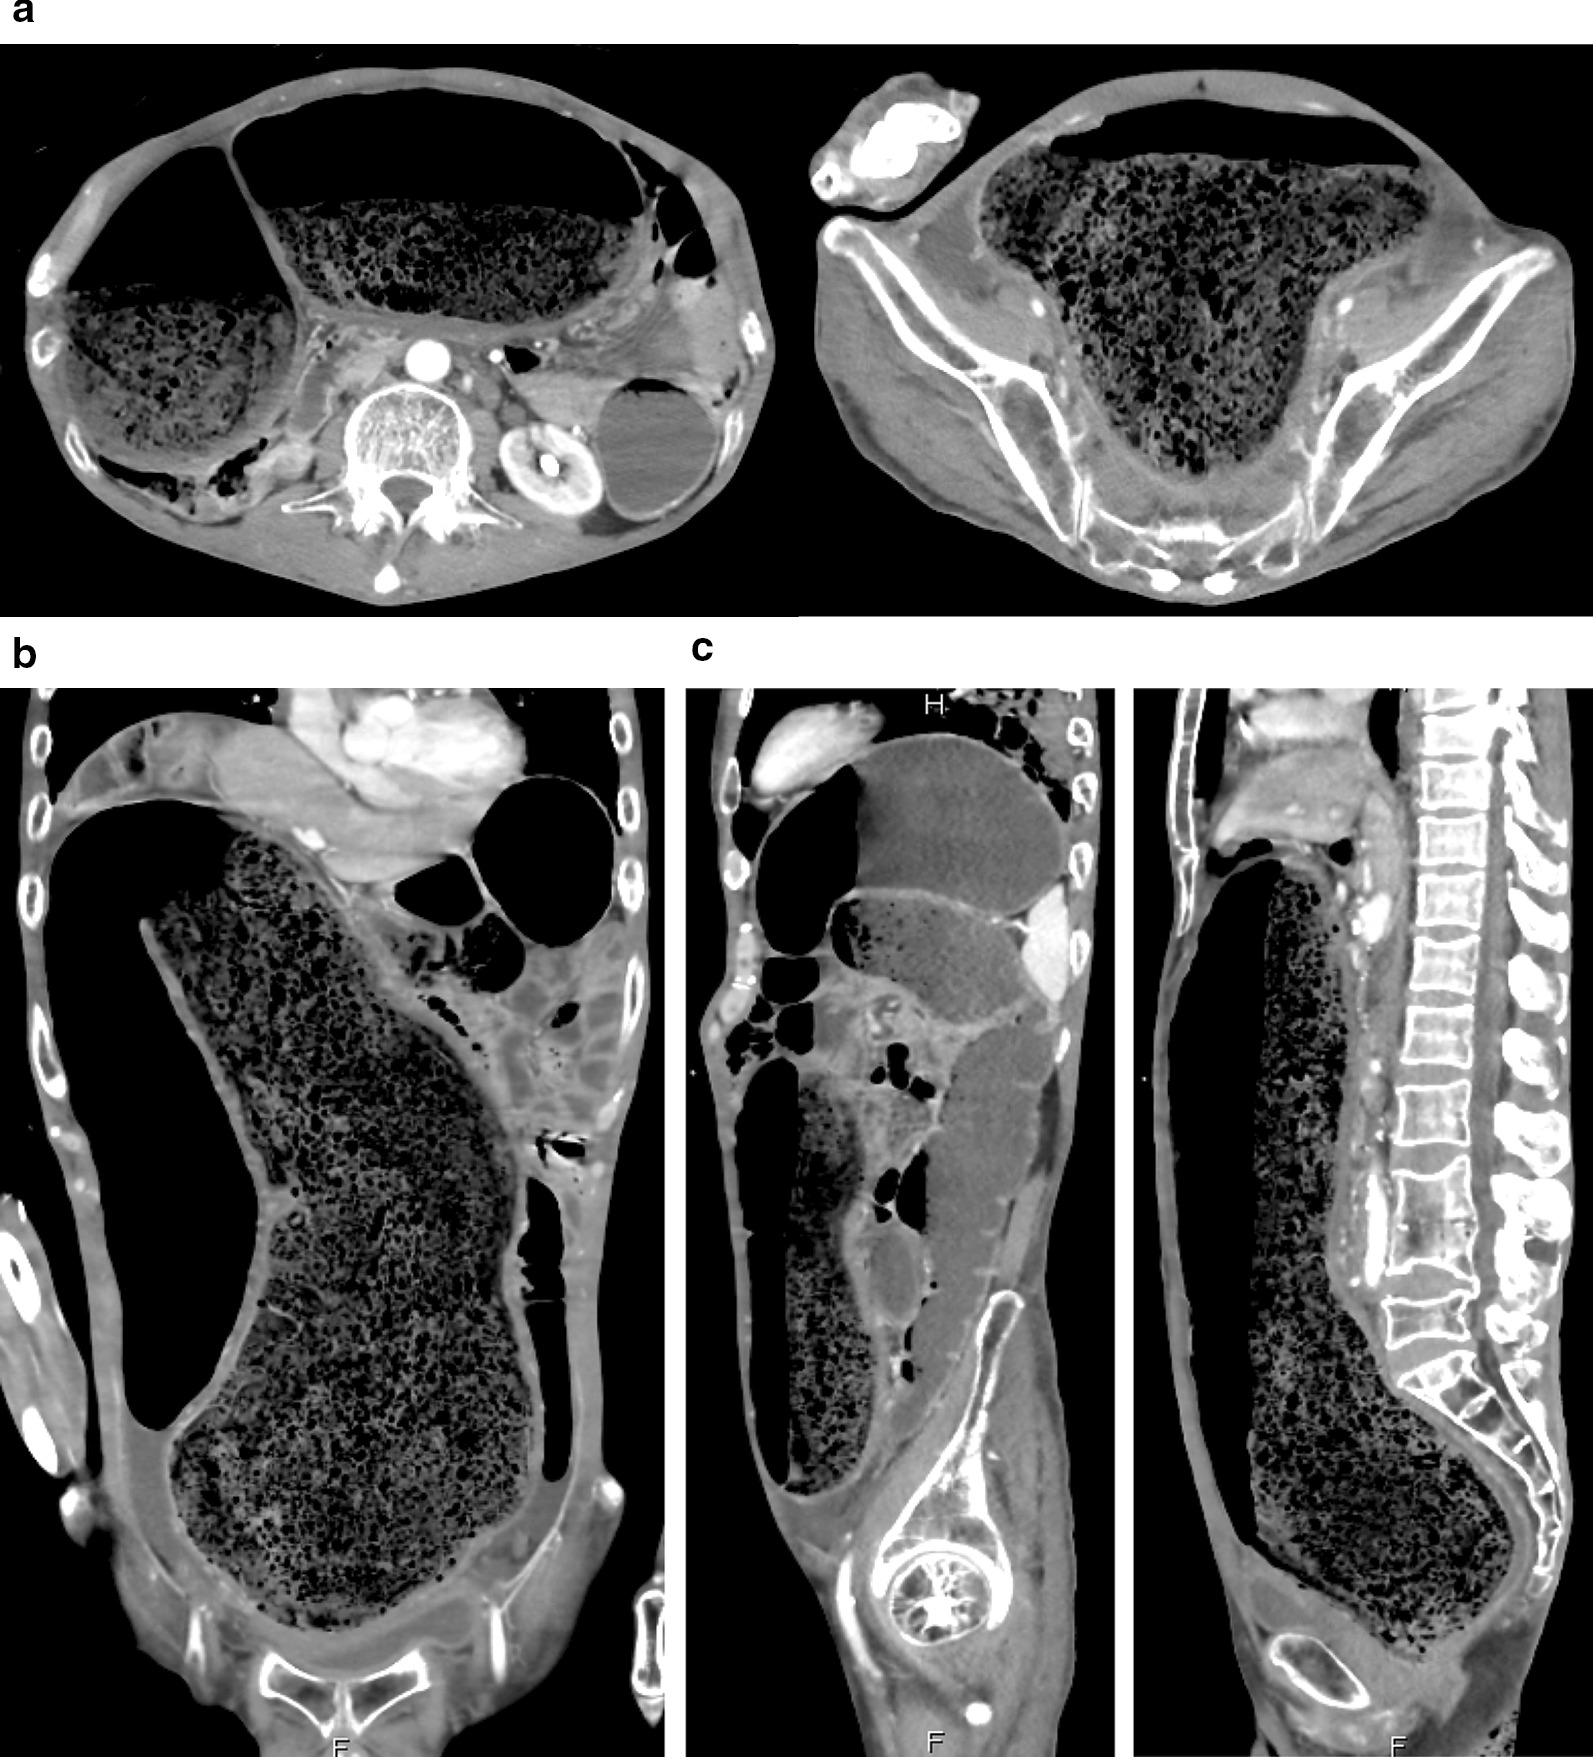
Arterial phase of abdominal dynamic computed tomography (a transverse plane, b coronal plane, c sagittal plane) on admission. Abdominal dynamic computed tomography of the abdomen revealed a thickened gut wall and extremely dilated sigmoid colon and rectum filled with a massive amount of stool as well as gas, compressing other intra-abdominal organs
